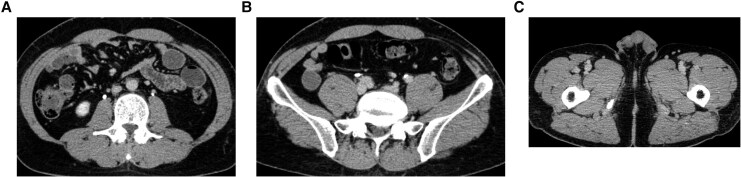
Computed tomography scans were obtained 7 weeks after removal of the inferior vena cava filter. (*A–C*) Images confirming complete resolution of thrombus (arrows).
